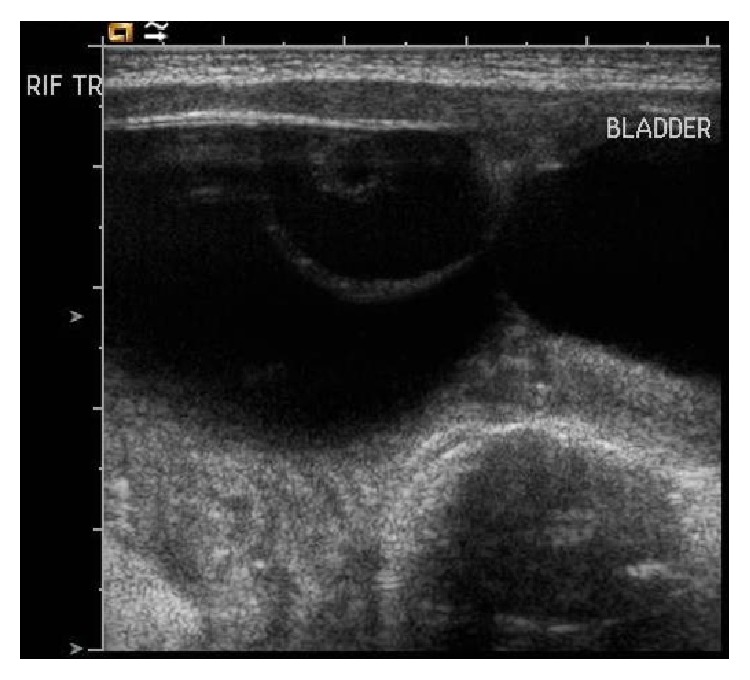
Abdomen and pelvic ultrasonography, showing a complex large cystic mass arising from right ovary approximately 7 cm in diameter. There is a 2 cm thin walled daughter cyst within the large cyst.
